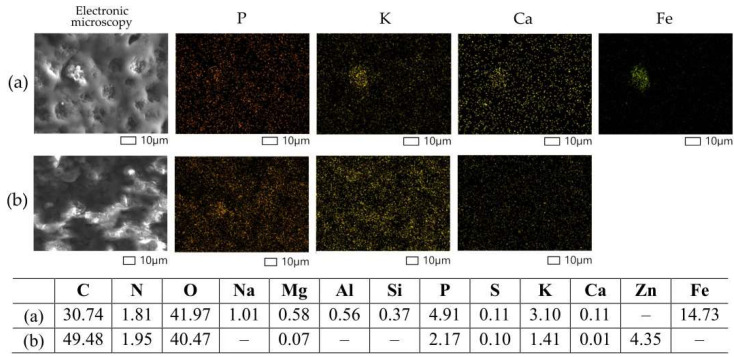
Elemental mapping and composition (mass %) of *R*. *rhodochrous* IEGM 1362 cells immobilized in PVA after 8 cycles with (a) and without (b) (–)-isopulegol determined using X-ray microanalysis. Distribution of individual chemical elements is highlighted with color.
